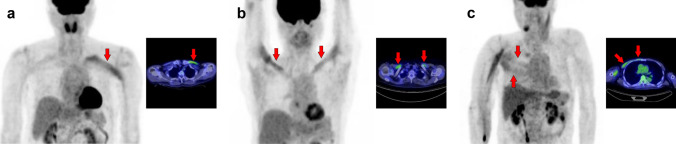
Pectoralis major muscle.* The pectoralis major generally consists of a clavicular part (a, b, arrows) and a sternal part (c, arrows); [^18^F]FDG uptake can be observed in both or separately. When the clavicular part is depicted alone, it may be misinterpreted as vascular inflammation
